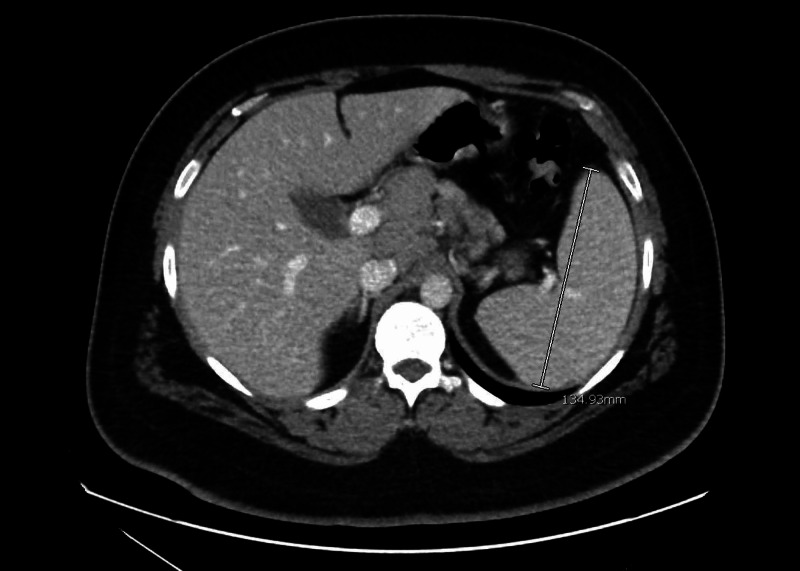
CT abdomen transverse section showing enlarged spleen measuring 13.49 cm in largest dimension (marked in white).
